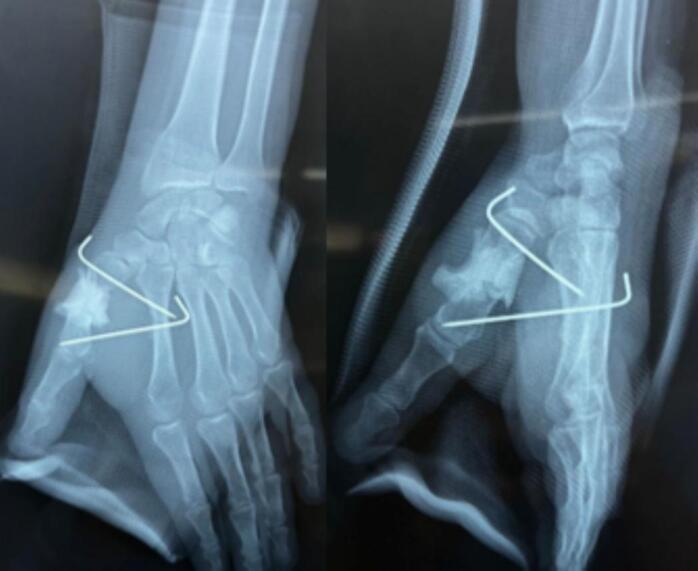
Postoperative radiograph of the first stage of the Masquelet procedure.
